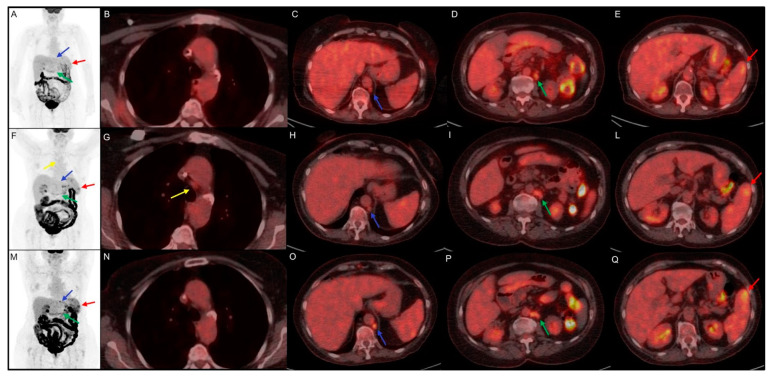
Clinical case of a 75 year-old female with history of classic Hodgkin Lymphoma firstly diagnosed in August 2014. She underwent chemotherapy with ABVD and, in September 2017, with brentuximab and R-bendamustine for disease relapse. In June 2019, the disease persistence induced hematologists to choose an off-label treatment with nivolumab. (A–E) 18F-FDG PET/CT performed before the start of immunotherapy, documented disease localized in para-aortic (blue arrow, SUVmax 4.5) and lombo-aortic (green arrow, SUVmax 5.5) lymph nodes and splenic lesions (red arrow, SUVmax 4.7). (F–L) Early 18F-FDG PET/CT performed 3 months after the start of the immunotreatment, indicate a dissociated response with decreasing SUVmax in para-aortic (blue arrow, SUVmax 2.5) and lombo-aortic (green arrow, SUVmax 4.5) lymph nodes but increased 18F-FDG uptake in splenic lesions (red arrow, SUVmax 5.7) and the appearance of a mediastinal adenopathy (yellow arrow, SUVmax 2.4). (M–Q) Interim 18F-FDG PET/CT, performed 6 months after the start of nivolumab, documented an increased uptake in para-aortic (blue arrow, SUVmax 6.9), lombo-aortic (green arrow, SUVmax 5.3) lymph nodes and in splenic lesions (red arrow, SUVmax 6.5). The mediastinal adenopathy previously detected showed no FDG uptake.
